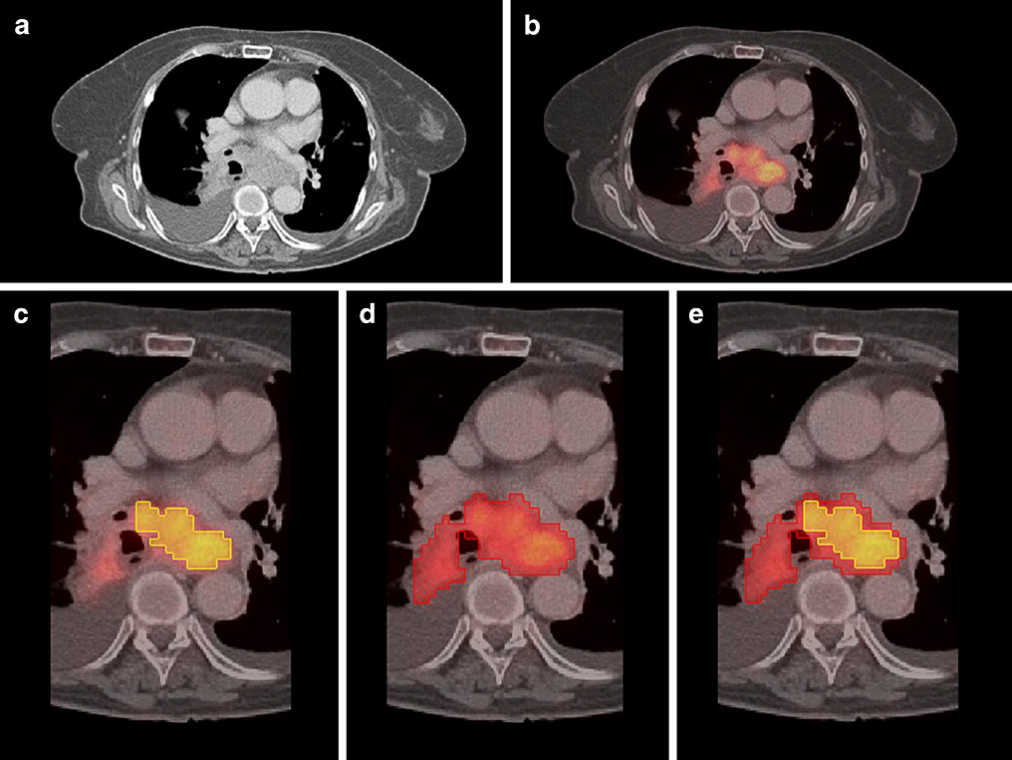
a CT alone, b fused [F18] FDG-PET/CT, c SUV30 semiautomated contour (*yellow*), d PERCIST semiautomated contour (*red*), e matched SUV30 (*yellow*) and PERCIST (*red*)
